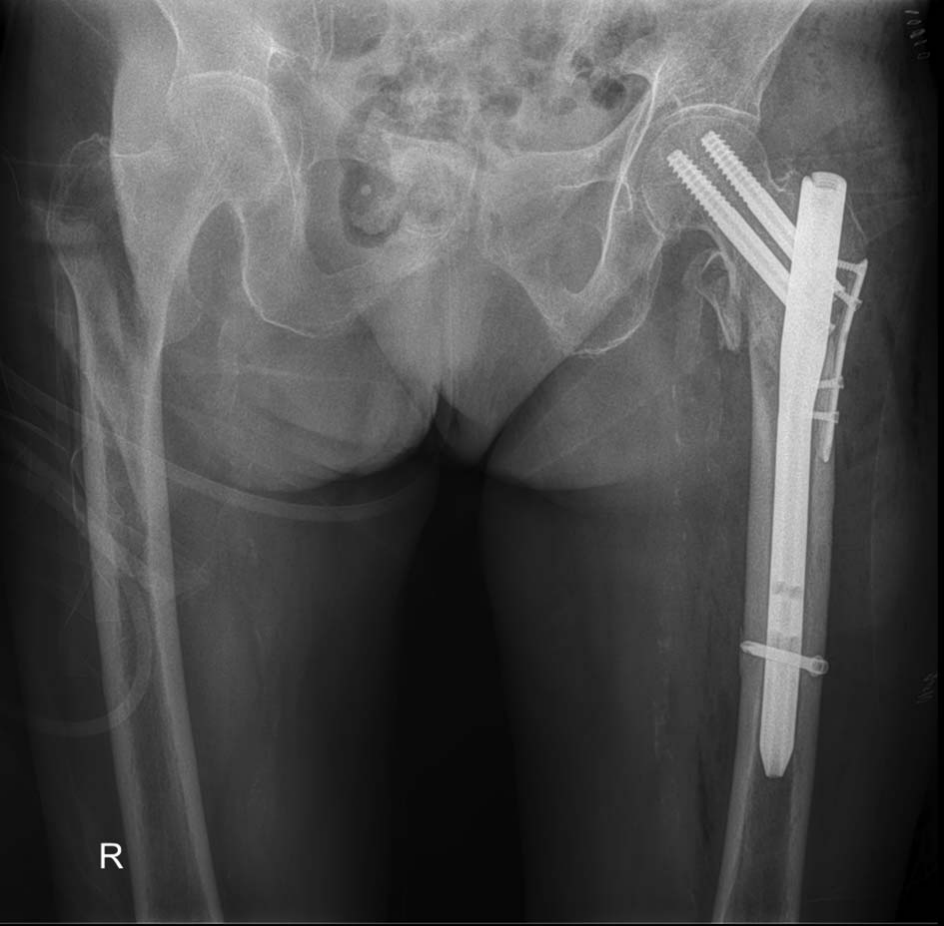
The postoperative radiographic appearance of a patient treated with a monocortical recon plate in combination with a proximal femoral nail.
